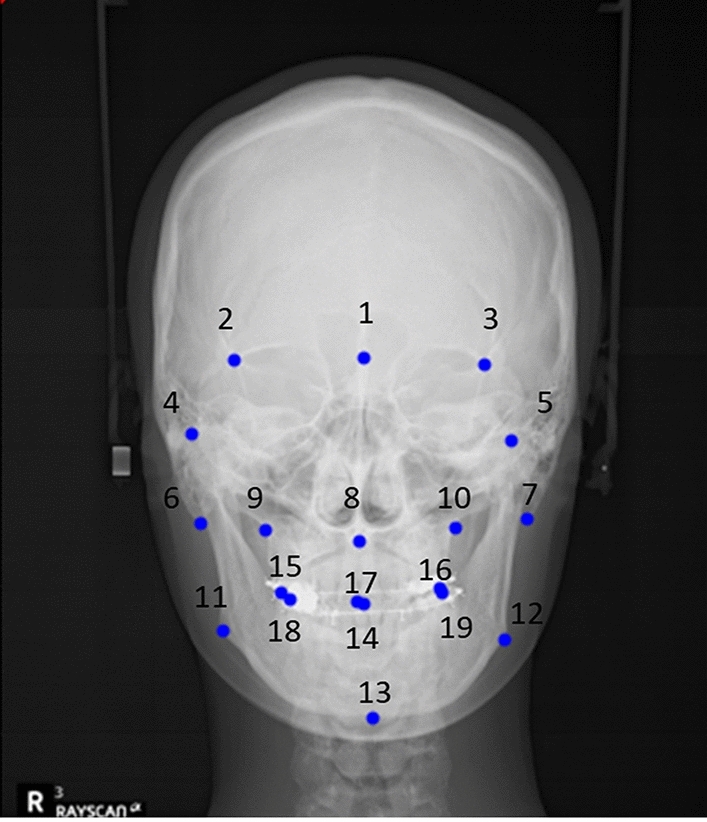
Landmarks on the PA cephalometric radiograph.
